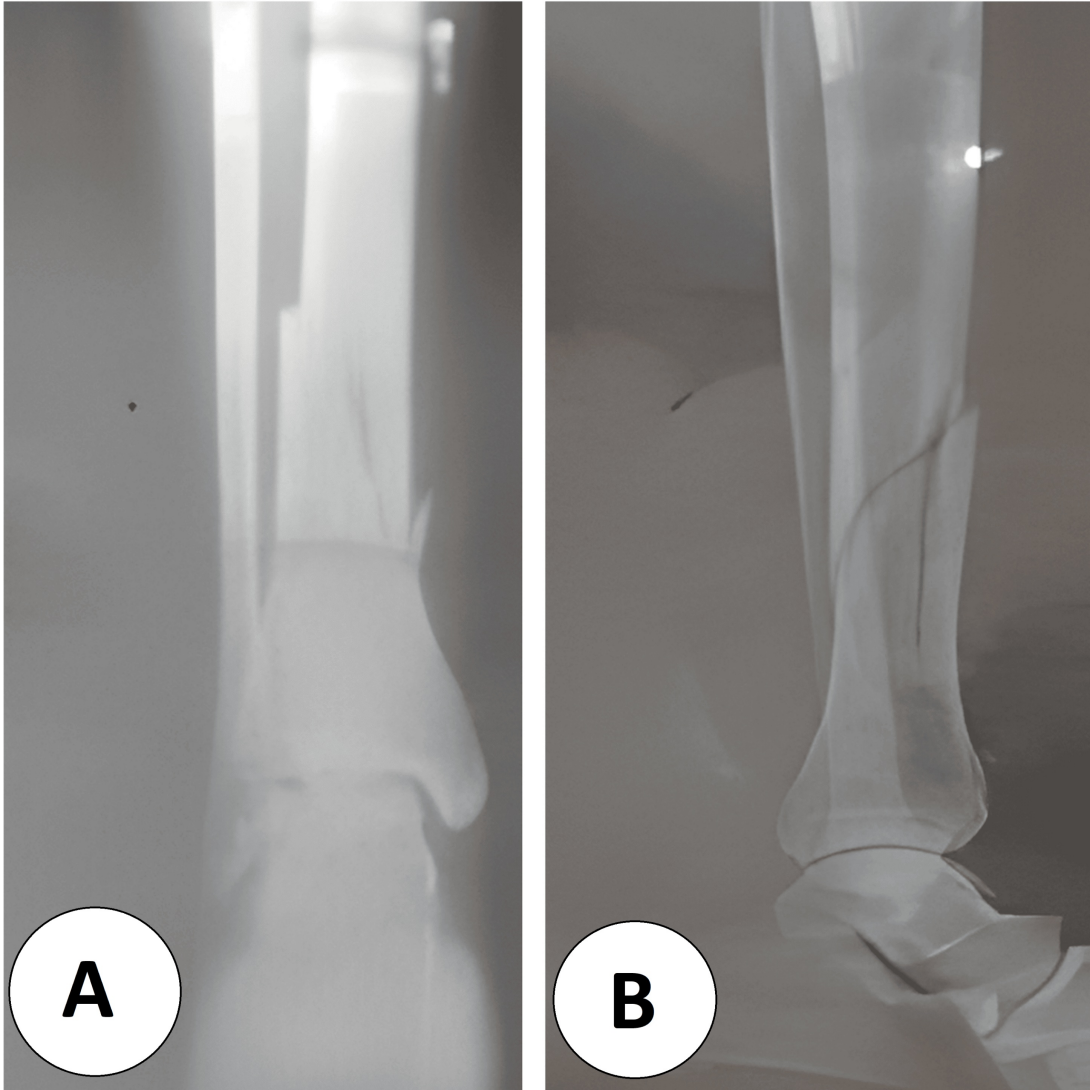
Preoperative injury radiographs (A) AP view showing an extra-articular distal-third tibial spiral/oblique fracture with metaphyseal comminution; ankle joint line preserved. (B) Lateral view demonstrating the long oblique fracture plane with a posterior spike and minimal displacement, without intra-articular extension.
